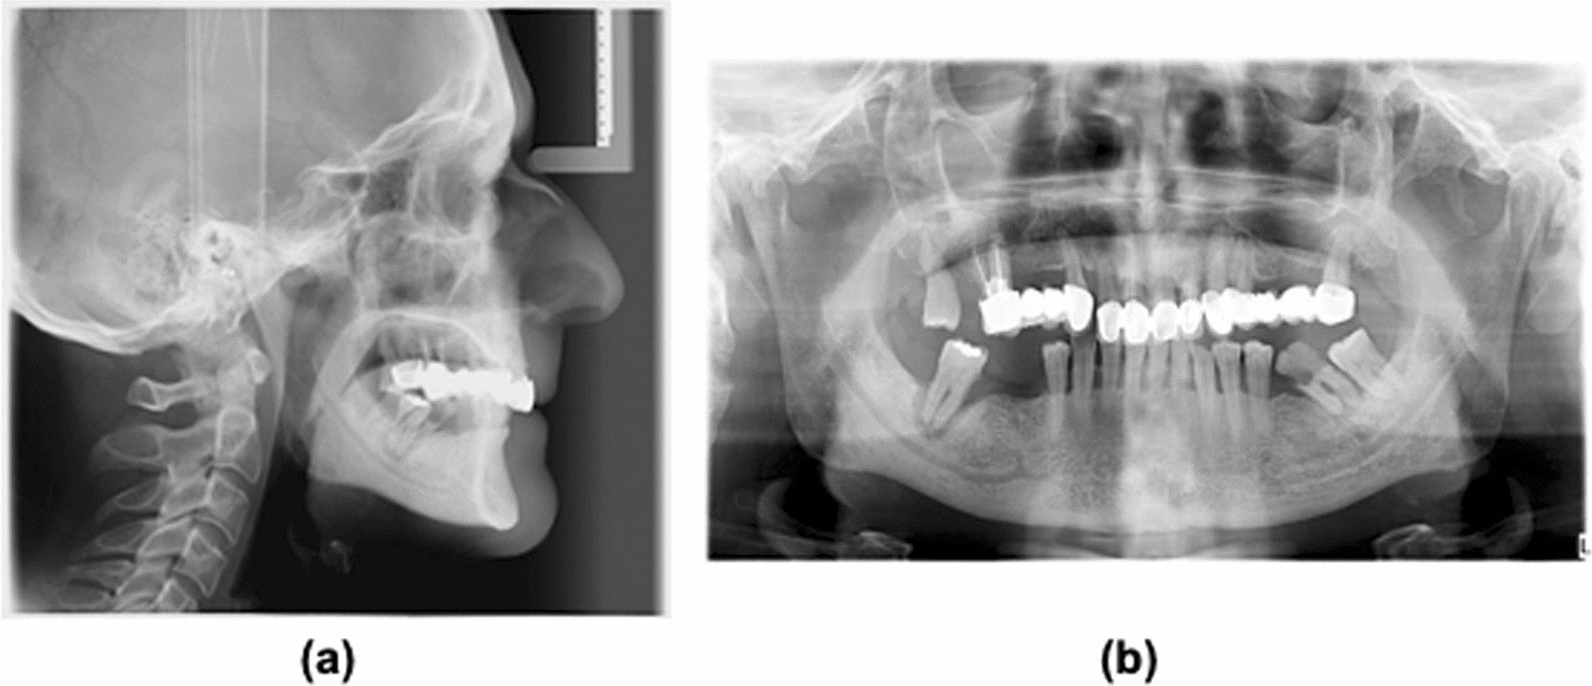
X–ray in lateral position (a) and Orthopantomography (b)
